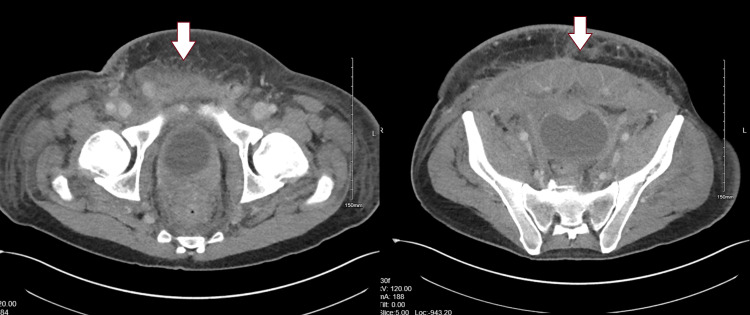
CT of the pelvis in the axial plane showing metastatic gastric adenocarcinoma along the anterior pelvic wall.
